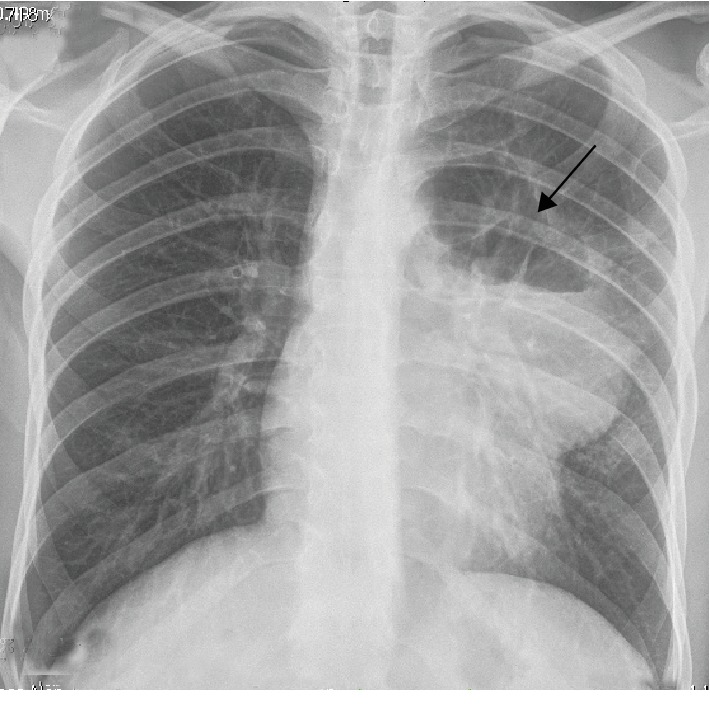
Male, 25-year-old patient with an anterior mediastinal mass (embryonal carcinoma germ cell tumor). Findings: cavitated mediastinal mass for the anterior segment of the left upper lobe (arrow). Technique: chest X-ray shows mediastinal mass.
